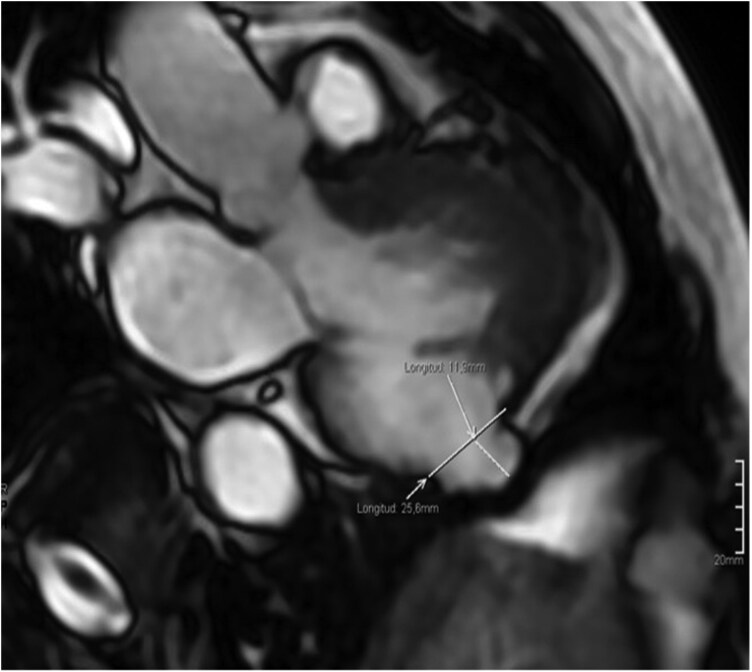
Cardiac MRI. Three-chamber view SSFP (Steady-State Free Precession) sequences or Cine. A large aneurysm in the basal and mid-inferolateral segments of the left ventricle with a saccular pseudoaneurysm (11 × 21 mm) at the aneurysm border.
